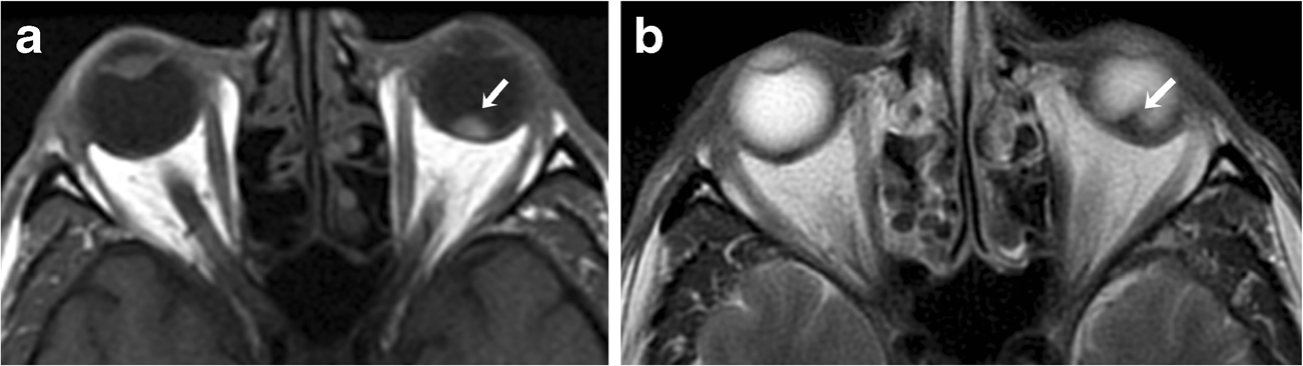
A 46-year-old male with uveal melanoma. a On the axial T1-weighted MR image of the brain, there is a T1 hyperintense choroidal mass (arrow). b The mass is hypointense on the corresponding axial T2-weighted MR image (arrow)
